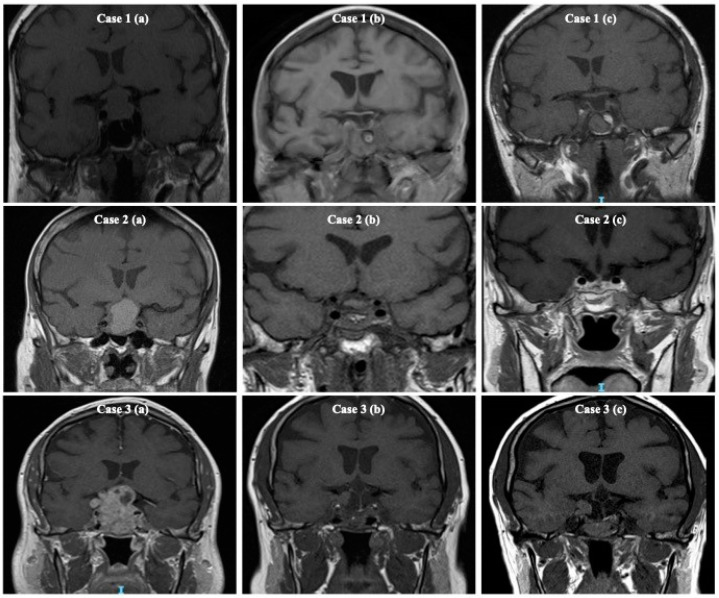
T1-weighted coronal Magnetic Resonance Imaging (MRI) scans demonstrating the degree of surgical excision in patients with non-functioning pituitary neuroendocrine tumours. The appearance of imaging is classified into three categories: complete resection, residual tumour, and sellar tissue of uncertain significance. These classifications are based on the visual appearance of the imaging results after the surgical procedure. In Case 1, the imaging protocol consisted of three distinct time points: (a) a preoperative MRI scan, (b) the first postoperative MRI scan indicating full excision, and (c) a follow-up MRI scan performed 5 years after the surgical intervention. In Case 2, the patient underwent a preoperative magnetic resonance imaging (MRI) scan (a). Subsequently, a first post-operative MRI was conducted (b) which revealed the presence of residual sellar tissue with undetermined relevance, as stated. Finally, a follow-up period of 5 years was observed after the surgery (c). In Case 3, (a) MRI scan before surgery, (b) the first postoperative MRI revealing evident residual disease, and (c) the 5-year follow-up MRI conducted after surgery. The image has been utilised in accordance with the permissions granted by Frontiers in Surgery [9].
